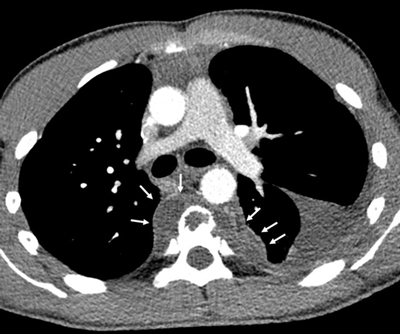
Contrast medium-enhanced axial CT scan of the chest (mediastinal window) at the pulmonary artery bifurcation level, showing the left pleural effusion (asterisk) as well as the pre-vertebral collections (arrows).
